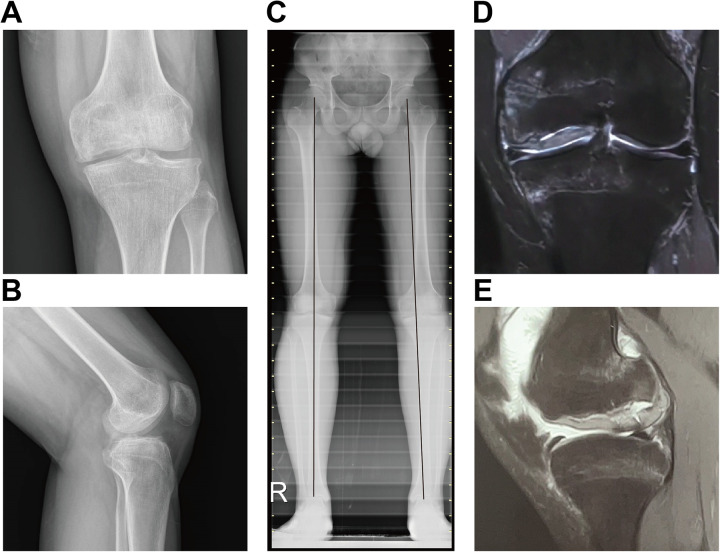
Preoperative x—ray, standing lower extremity radiograph, and magnetic resonance imaging (MRI). (A,B) Plain radiographs of the left knee showed an obvious osteochondral lesion with a large bony defect in the MFC and narrowing of the medial joint space. (C) Standing lower extremity radiograph showing slight valgus of the left knee. (D,E) Preoperative MRI shows the osteochondral lesion in the posterior aspect of the left MFC in the coronal and sagittal planes.
